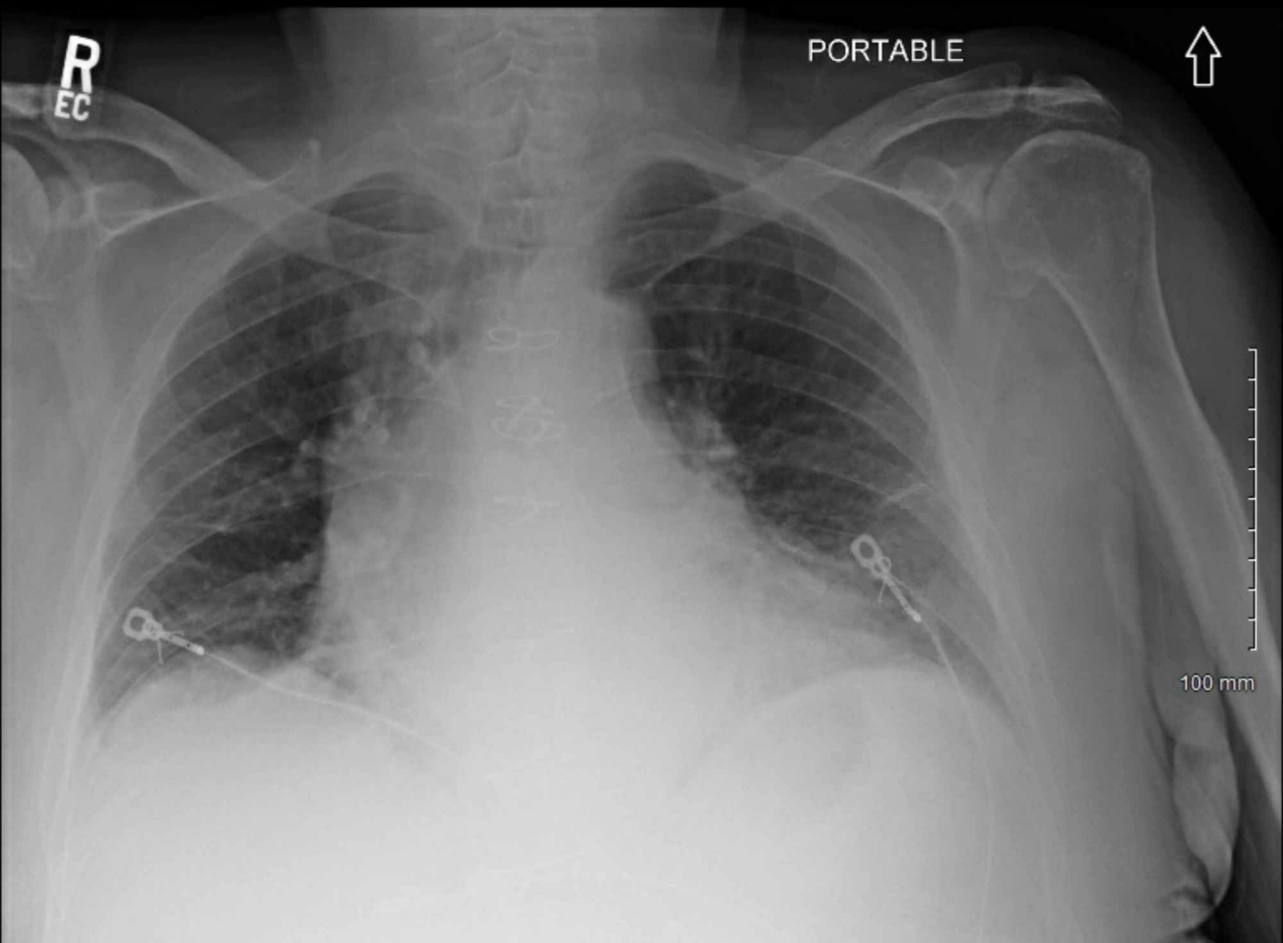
Chest x-ray Chest x-ray consistent with pulmonary edema and trace right pleural effusion.
